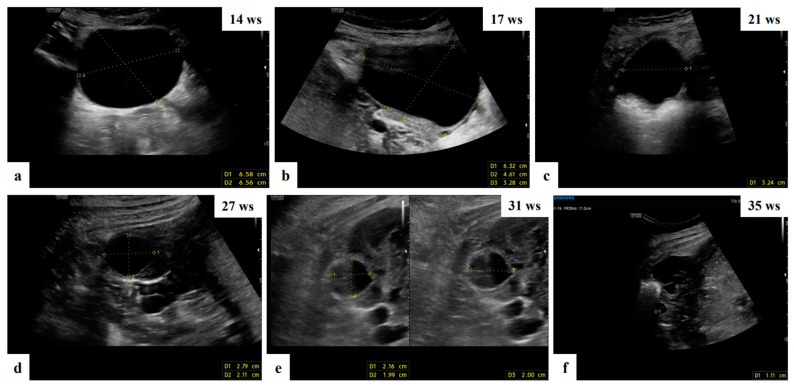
Grayscale ultrasound images showing adnexal anechoic unilocular mass suspected of functional cyst in ultrasound of 32 ys old patient managed expectantly during pregnancy who attended a monthly scan and had a reduction in the cyst dimension compared to the initial measurement and complete resolution at 35 weeks of gestation (a–f).
